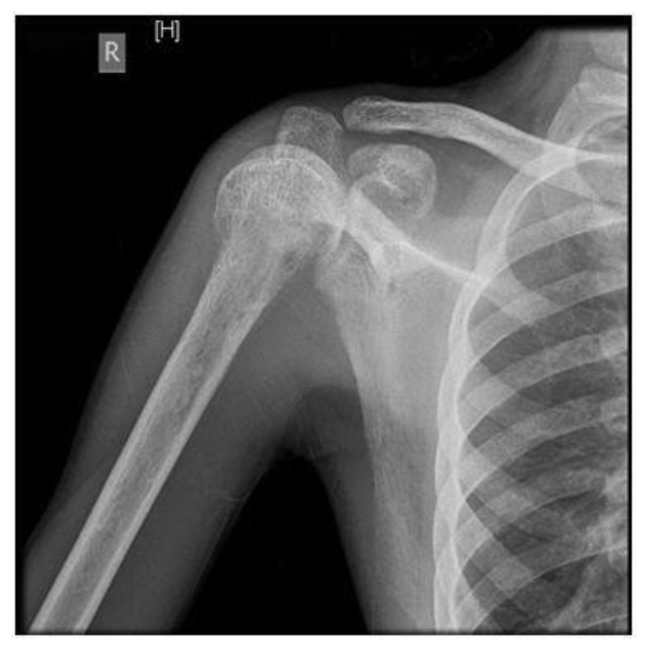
Xray right shoulder AP view showing irregular permeative type of lytic lesions involving proximal metadiaphysis region of the right humerus, cortical breaks, interrupted periosteal reaction and a wide zone of transition. No significant soft tissue component is present.
